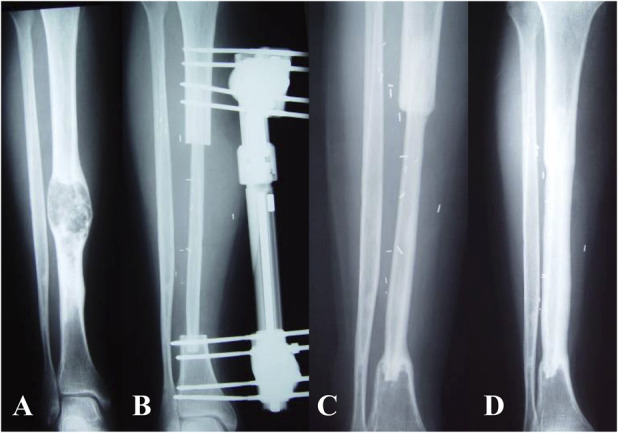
(A) This radiograph shows a diaphyseal osteoblastic osteosarcoma in a 19-year-old man. (B) The patient underwent intercalary resection of the tibia and reconstruction with VFG and external fixation. (C) After union of both osteotomies was achieved at 6 months after the index surgery, the external fixator was removed. (D) This radiograph shows progressive hypertrophy and remodeling of the vascularized fibula under mechanical stress once hardware was removed.
